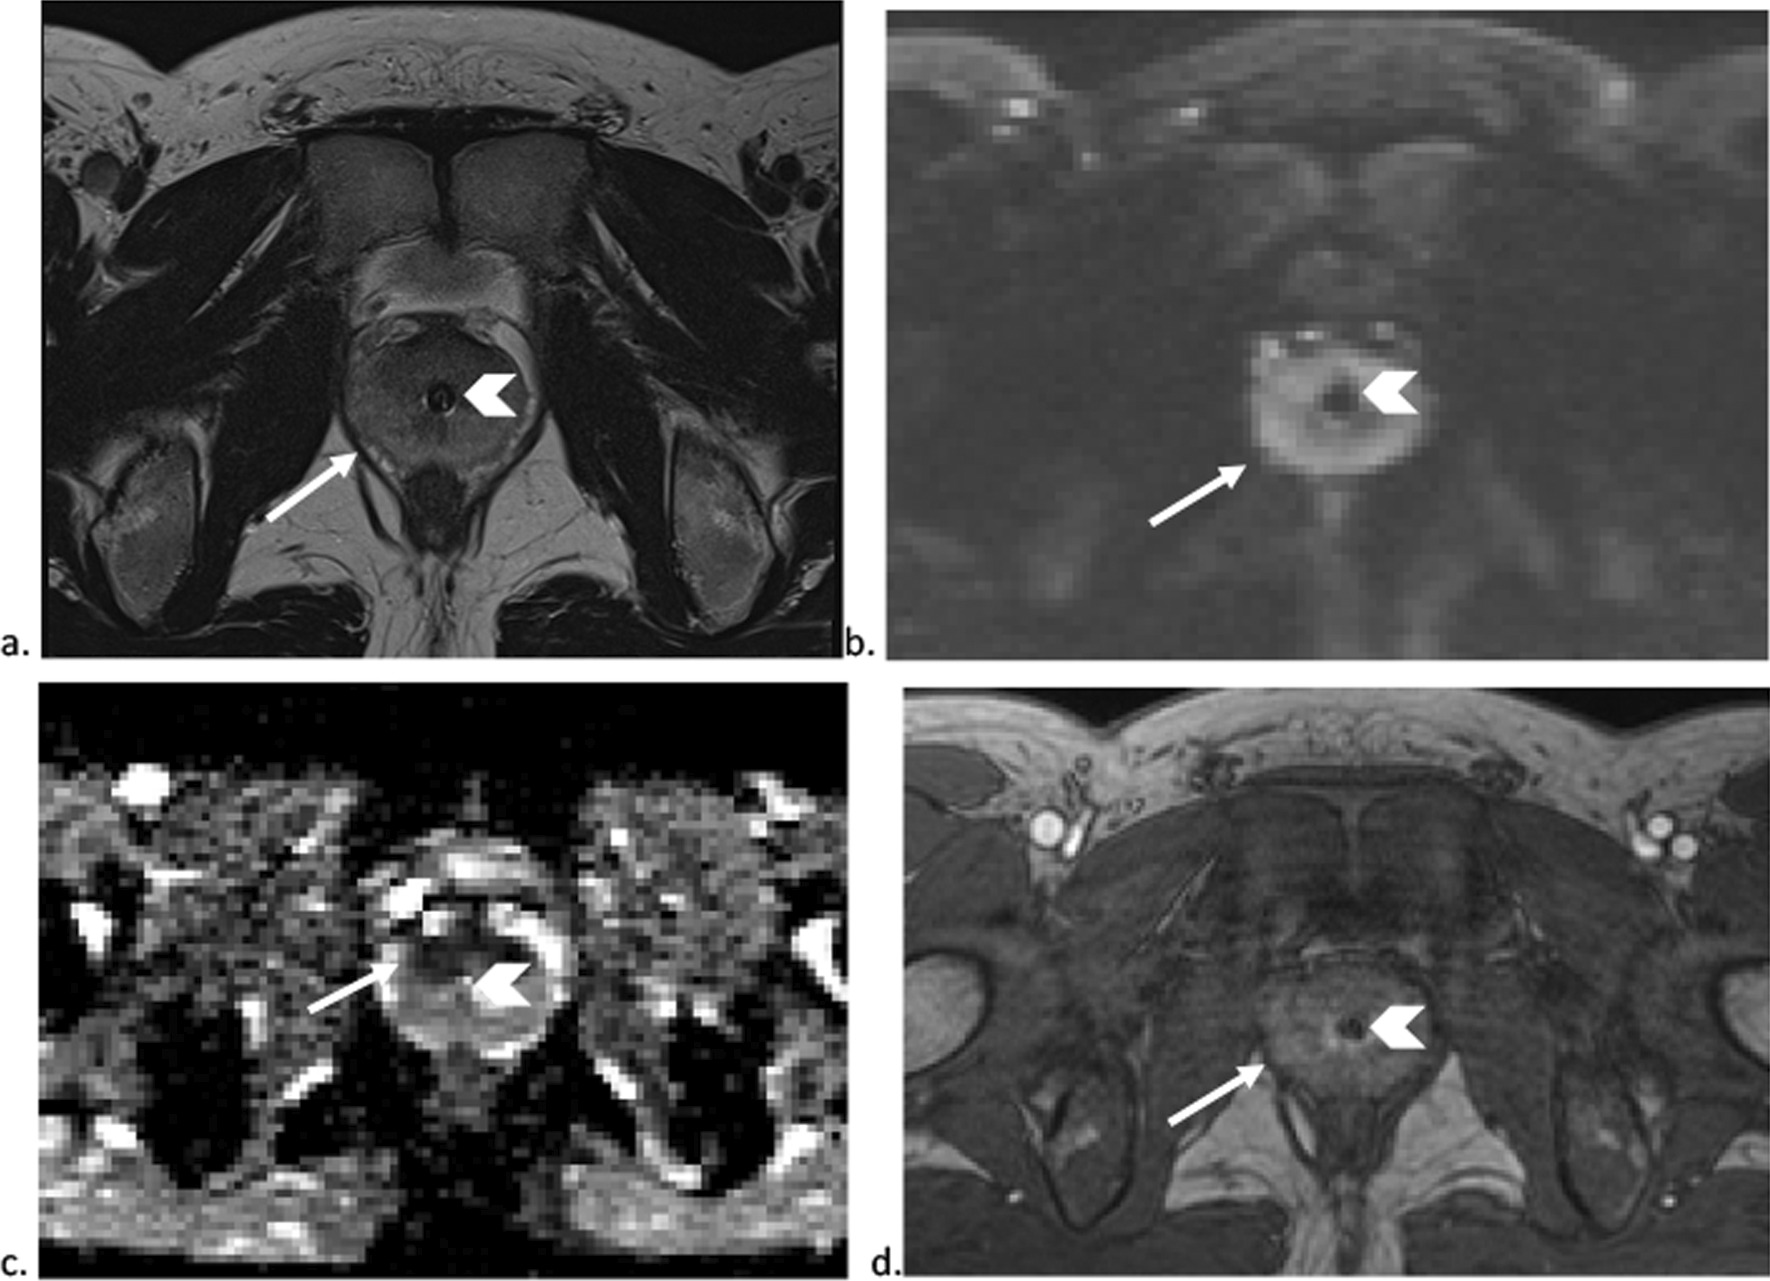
MRI of the prostate gland showing diffuse prostatitis. T2 weighted (a), high b value 1500 DWI (b), ADC map (c) and dynamic contrast-enhanced (d) images show the prostate gland with a volume of 30 cc with a Foley catheter in situ (arrowhead). The peripheral zone is diffusely mildly T2 hypointense (a) (arrow). There is diffuse restricted diffusion (b, c), which is greater in the transition zone with lower signal intensity on the ADC map (c) (arrow). There is diffuse mild (d) hypervascularity (arrow)
